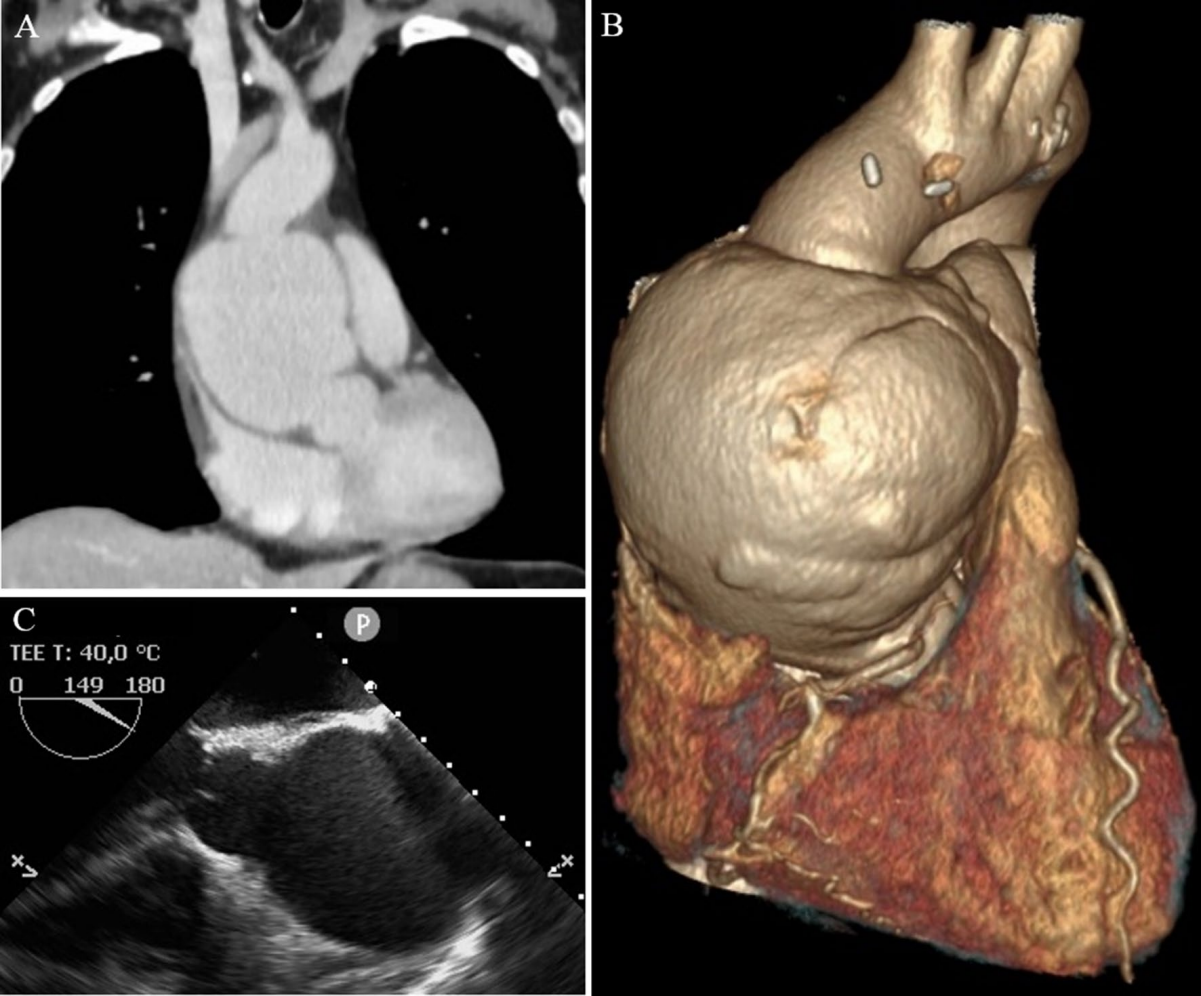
Perioperative imaging of the ascending aortic pseudoaneurysm. Coronal plane of CT scan (A) and three-dimensional CT reconstruction (B) showed a huge, saccular dilatation of the tubular portion of ascending aorta. This abnormality could be also visualised by intraoperative transesophageal echocardiography (C)
